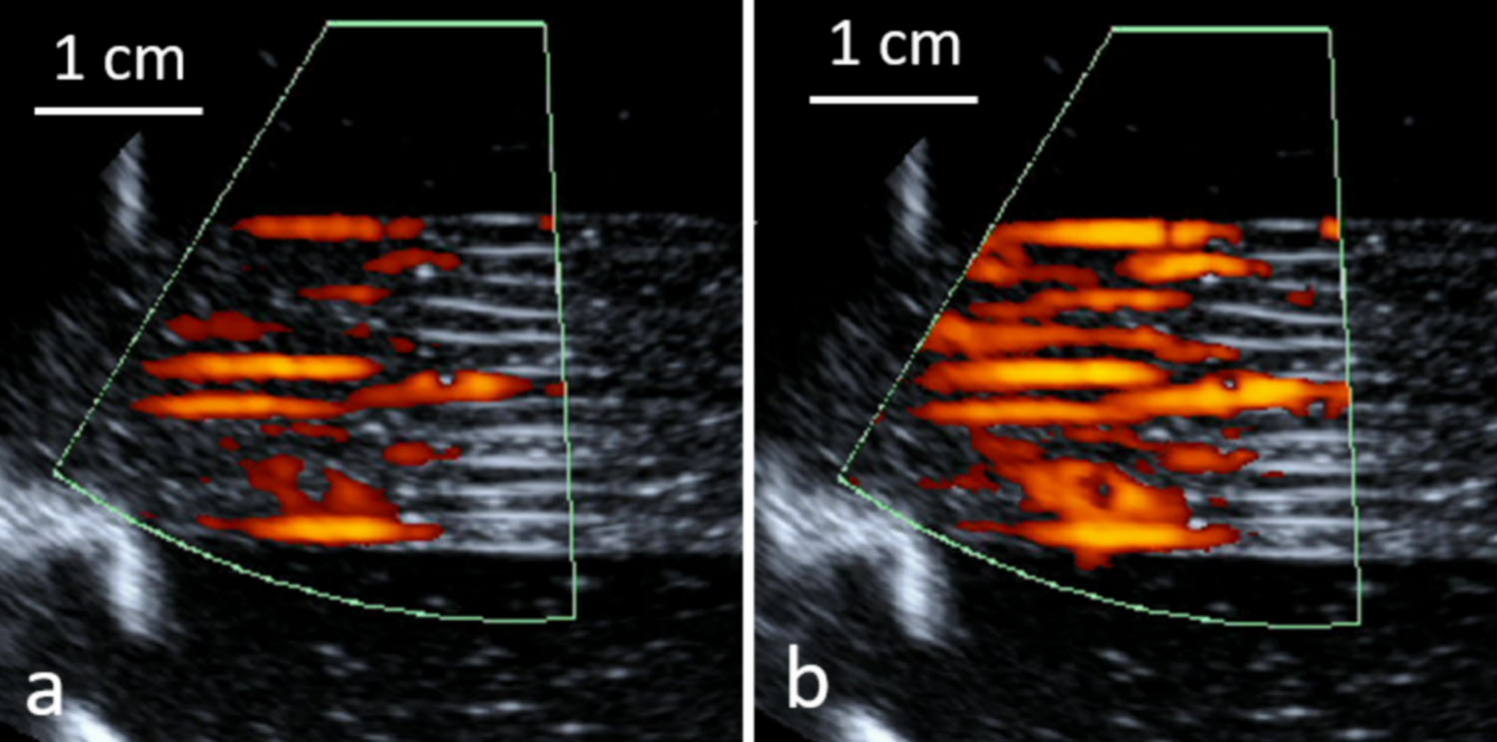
Laminar flow of the in vitro model of perfused tissue observed by pulsed color Doppler ultrasonography during a phase of ‘diastole’ (a) and ‘systole’ (b). Carrier frequency 5 MHz, pulse repetition frequency 100 Hz.
